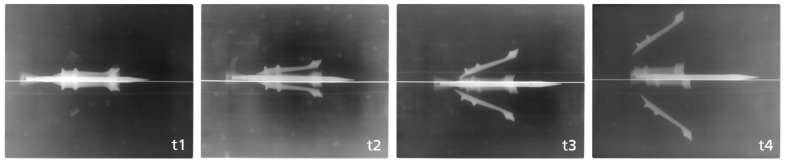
Example of the X-ray images for one orientation and all four positions (t1–t4). The first image at the point of time t1 is positioned 500 mm behind the muzzle; hence, t2 is at 1500 mm, t3 at 2500 mm and t4 at 3500 mm.
